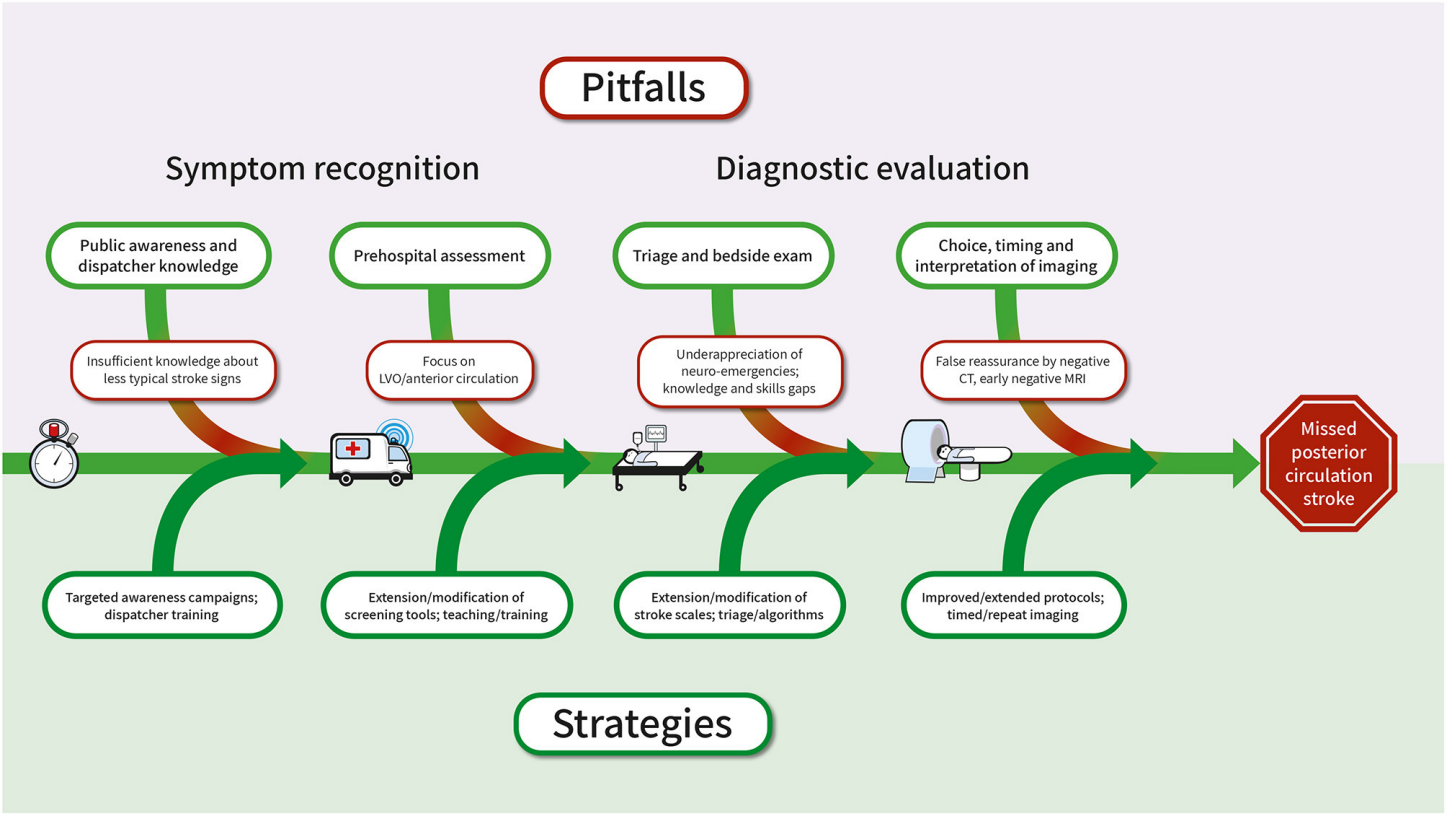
Pitfalls associated with the diagnosis of PCS in the chain of acute stroke care and suggested approaches to solution. CT, computed tomography; MRI, magnetic resonance imaging; PCS, posterior circulation stroke.
